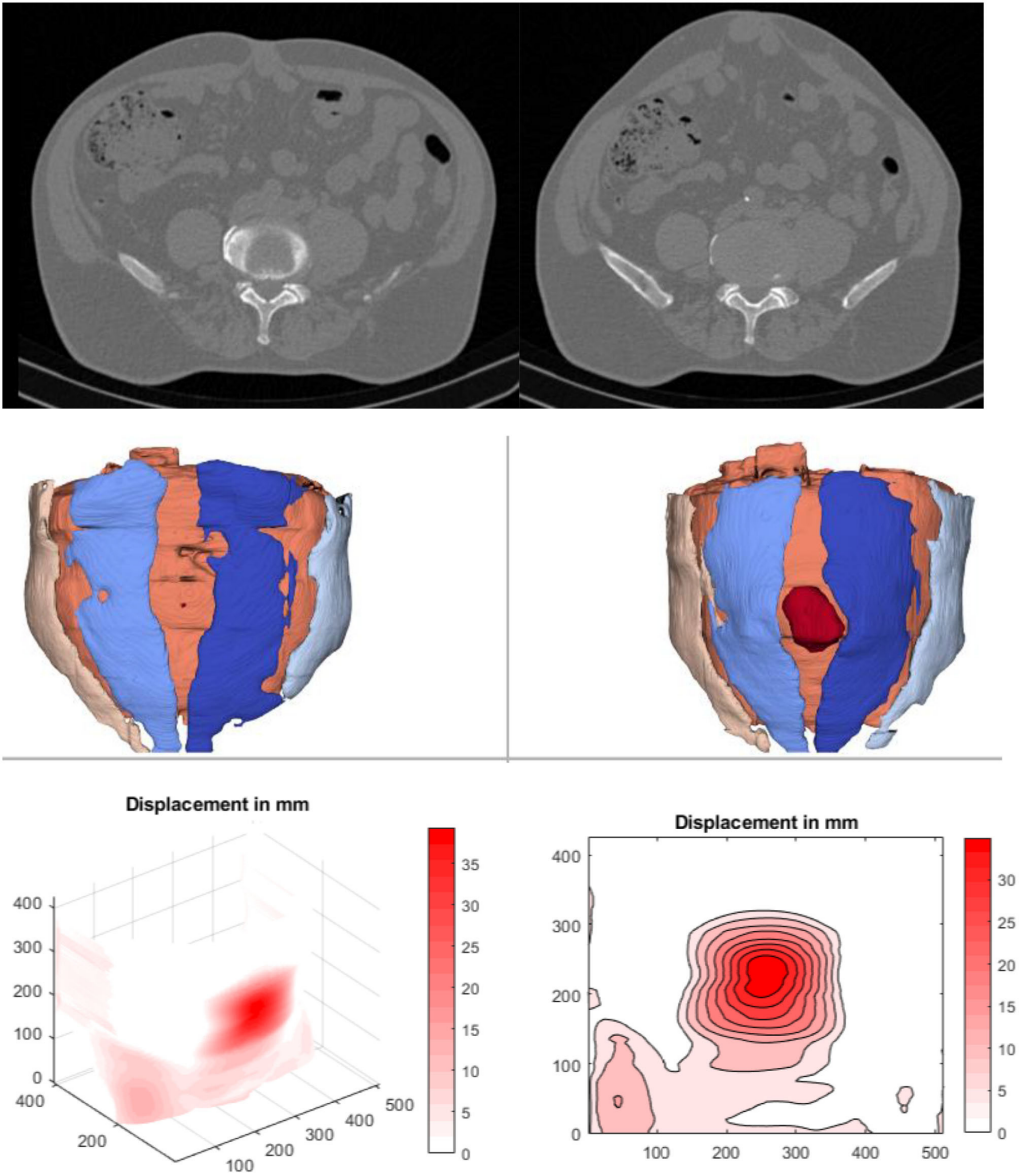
Imaging of a male, 50–55 years old, with an incisional hernia after an umbilical hernia repair. Top: A single slice of an abdominal CT scan at rest (left) und during a Valsalva maneuver (right) after the slice recognition of the DICOM data using 3D Slicer^®^. It is obvious that load deforms not only the median hernia but the entire abdominal wall. Middle: Result of the automatic segmentation using the artificial intelligence described above. At rest, the surface does not bulge due to a missing protrusion (left). Once the protrusion gets pronounced during a Valsalva's maneuver, the hernia becomes obvious (red volume in the right middle panel). The rectus musculature is depicted light and dark blue to the right and to the left of the hernia orifice. Between the musculature, the linea alba is lightly cream colored and thinned out to a plane. Lower panel: A surface projection of the tissue displacement upon Valsalva (left) and an isodistance mapping of the superficially visible displacement fields (right)—closely adjacent lines show high gradients and thus main stress zones. The center of the hernia opening in the evaluation is colored darkest red indicating a displacement of almost 4 cm.
